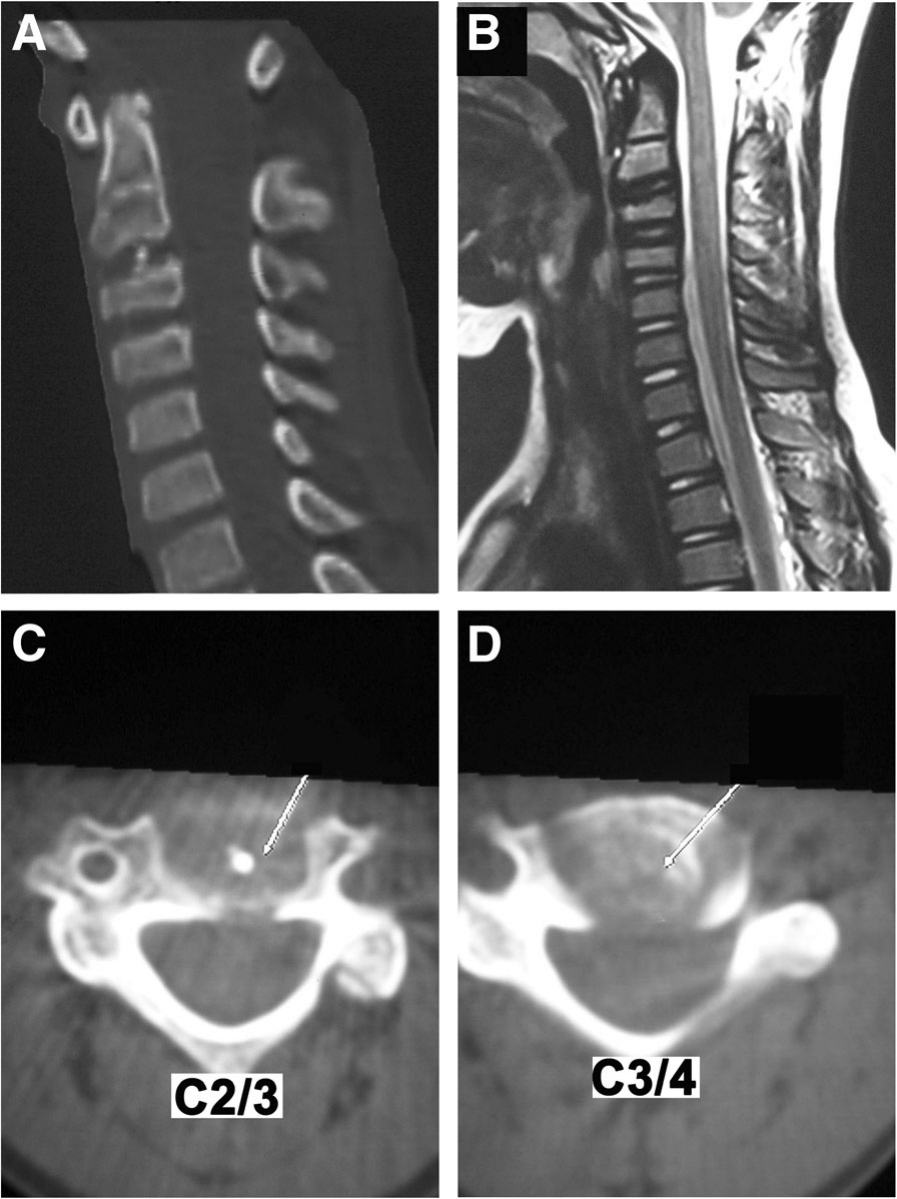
Radiological imaging at 19-month follow-up. a Computed tomography revealed the IDC and OPLL at the C3/4 level has disappeared, only minor calcification at C2/3 intervertebral disc left. b Magnetic resonance imaging revealed restoration of T2-weighted signal intensity of C2/3 and C3/4 discs. c Axial computed tomography through C2/3 revealed minor calcification at C2/3 intervertebral disc left. d Axial computed tomography through C3/4 revealed IDC and OPLL at the C3/4 level disappeared. (Adopted and reedited from Du et al. [3] with permissions of all authors)
